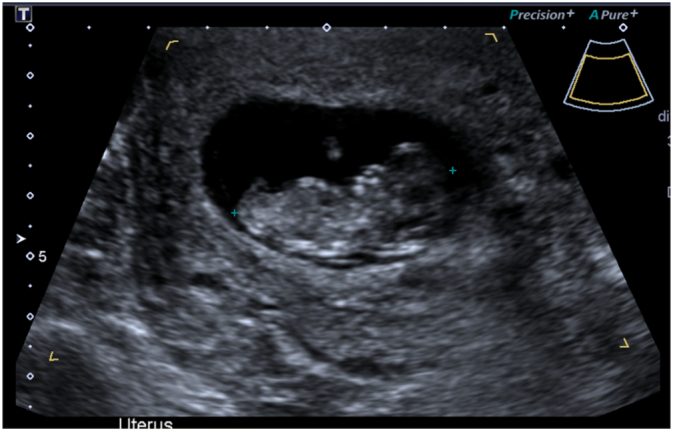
Ultrasound showing live intrauterine foetus.
